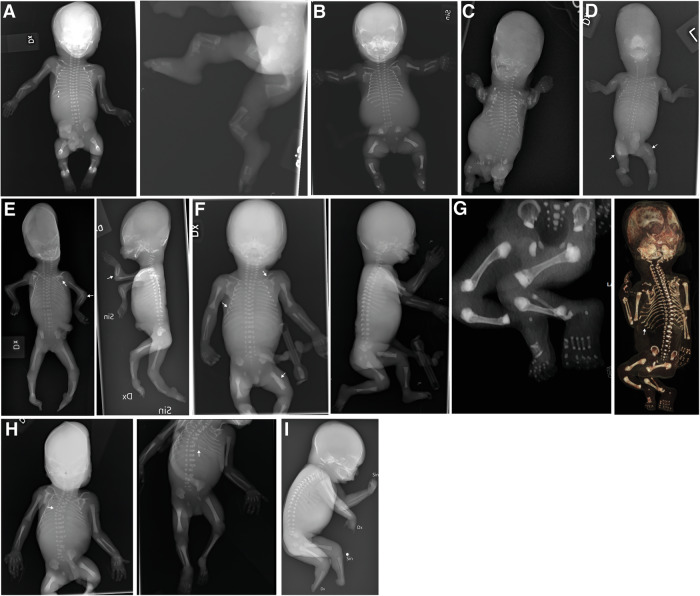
Skeletal radiographs of selected fetuses in the study with rare phenotypes and unsolved molecular diagnoses. A Fetus 20, 19 weeks. OI type 5, *IFITM5*-related (OMIM#610967). Note mildly reduced mineralization with several bilateral rib and left clavicle fractures (arrows), some with callus formation. Steep angulation of long tubular bones, except for humerus. B Fetus 22, 20+ weeks. Opsismodysplasia, *INPPL1*-related (OMIM#258480). Note severe platyspondyly, iliac hypoplasia, short tubular bones with irregular metaphyses and remarkable brachydactyly in hands and feet. C Fetus 23, 13 weeks. Greenberg dysplasia, *LBR*-related (OMIM#215140). Note irregular mineralization. Severely short long tubular bones with anarchic ossification. Thorax hypoplasia with gracile irregular ribs. Low mineralization of vertebral bodies. D Fetus 25, 14 weeks. Cole-Carpenter syndrome, *SEC24D*-related (OMIM#616294). Note low mineralization, short extremities and multiple fractures of the ribs and tubular bones (arrows). E Fetus 26, 16 weeks. Acrofacial dysostosis, *SF3B4*-related (Nager syndrome OMIM#154400). Note retromicrognathia, left scapular hypoplasia (arrow), elbow misalignment, bilateral radioulnar synostosis (arrows), ulnar deviation in wrists, bilateral absence of thumbs, bent tibia, right fibular hypoplasia, left fibular aplasia, and bilateral pes equinovarus. F Fetus 29, 18 weeks. OI, unsolved molecular diagnosis. Note decreased mineralization of skull and face. Left femoral fracture with callus (arrow). Few rib and left clavicular fractures (arrows). Markedly slender diaphyses of the long tubular bones. G Fetus 30, 17 weeks. Metaphyseal osteosclerosis, unsolved molecular diagnosis. Postmortem CT. Note severe sclerosis of the metaphyses in tubular bones, metaphyseal equivalents and in vertebral bodies. Slender ribs with bilateral fractures (arrow). H Fetus 31, 20 + 1 weeks. Spondylocostal dysostosis, unsolved molecular diagnosis. Note multiple segmentation anomalies (arrow), leftsided partial dorsal rib fusions (costae 6–7 and 8–10) (arrow), and unilateral left acetabular dysplasia. I Fetus 32, 20 weeks. Peromelia/ectrodactyly, unsolved molecular diagnosis. Note reduction anomalies of all four extremities. Absent feet. Left hand with shortened fingers 2–4 and partial syndactyly, right hand with shortened fingers 1 and 5, and stumps of fingers 2–4.
